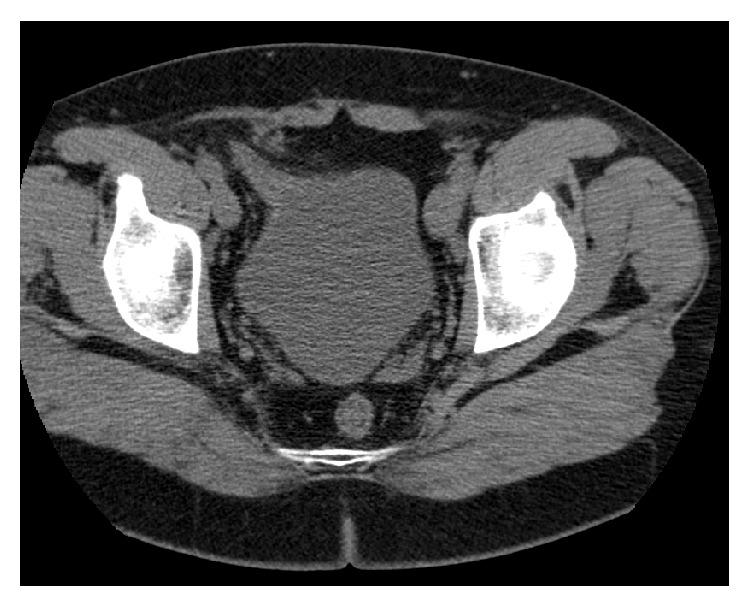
Noncontrast CT: right inguinal hernia containing the bladder wall.
